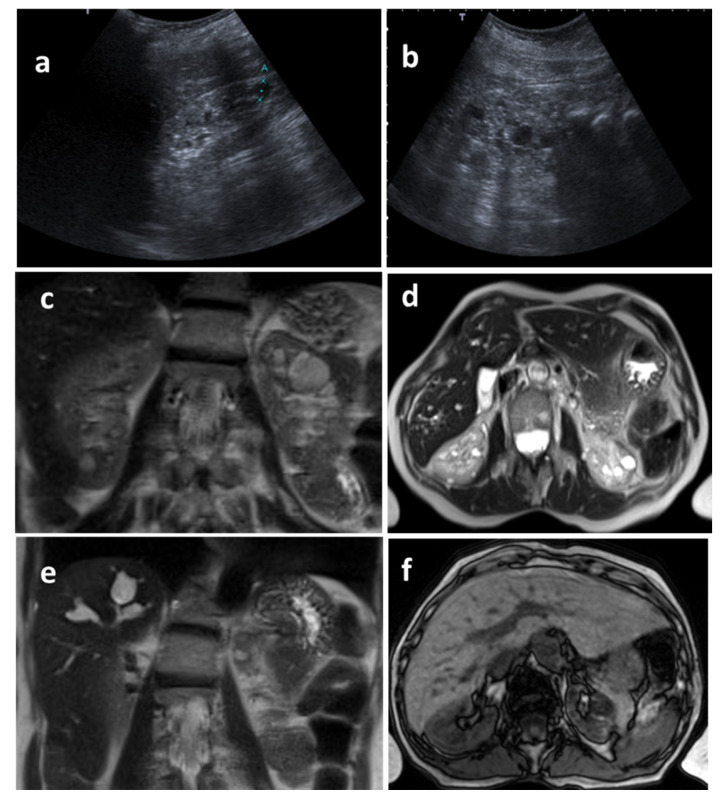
Image analysis of the index case. (a,b) Renal ultrasound, longitudinal view. The kidneys show preserved parenchymal thickness, although with markedly increased echogenicity and loss of corticomedullary differentiation. Multiple cystic images and parenchymal calcifications are observed. (c,d) MRI, coronal and axial sections, demonstrating multiple renal cysts resulting in an irregular renal contour. (e,f) MRI, coronal and axial sections, showing saccular and fusiform dilatations of the intrahepatic biliary tree.
